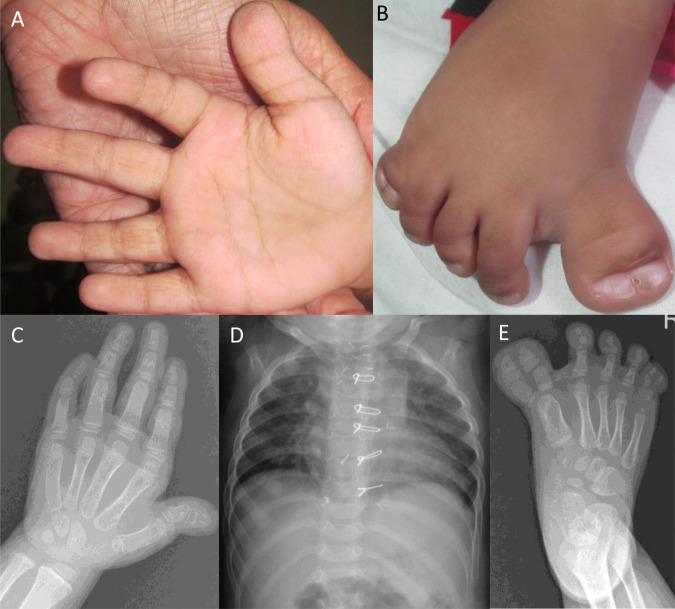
Clinical features of proband 1 at age 2 years and 9 months. Short and broad thumbs and clinodactyly of 5th finger were noted (A and C). Preaxial and postaxial polysyndactyly were observed in the right foot (B and E). Chest radiograph is unremarkable (D).
